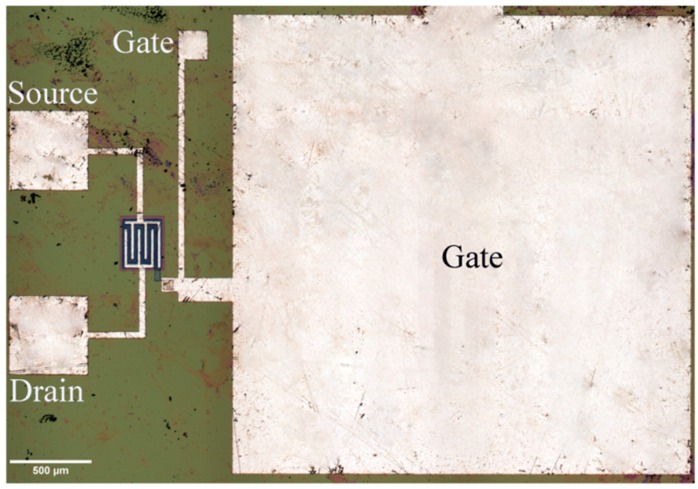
One of the first examples of an EGFET-based sensor realized in the 1980s. It is characterized by an aspect ratio of 1900/5 μm/μm, originally fabricated for an integrated ultrasonic transducer, resulting from a collaboration with the Center for Sensor Technologies, University of Pennsylvania (courtesy of Prof. J. Van der Spiegel) [14].
